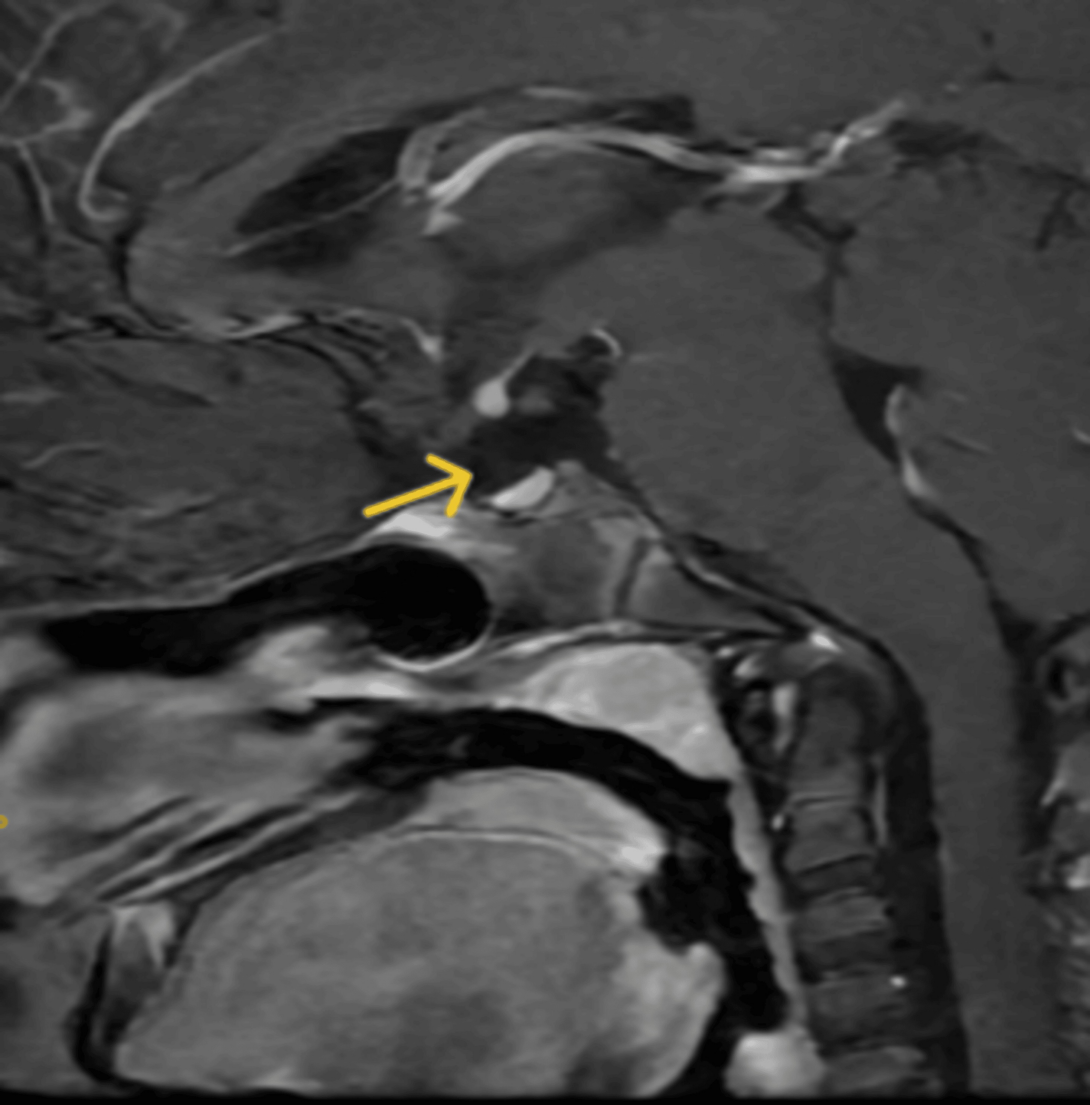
Sagittal T1-weighted contrast-enhanced magnetic resonance imaging of the sella turcica shows absent pituitary infundibulum (yellow arrow) in Case 1
